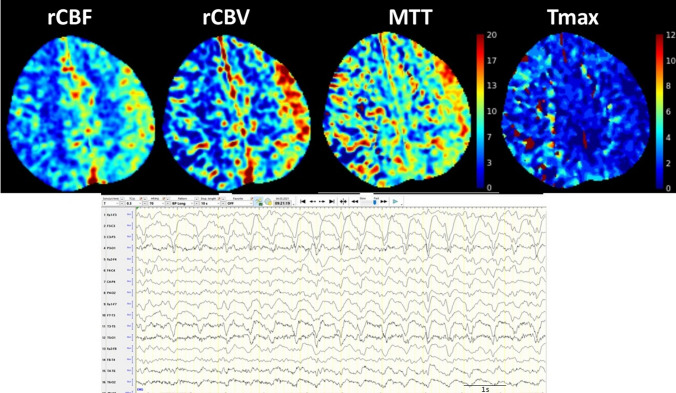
A 78-year-old patient with a history of structural epilepsy following a left middle cerebral artery (MCA) ischemic stroke presented with tonic–clonic status epilepticus, followed by aphasia and right-sided hemiparesis. Computed tomography perfusion (CTP) imaging demonstrated hyperperfusion localized to the anterior, lateral, and posterior MCA territories on the left, with increased cerebral blood volume (CBV) and cerebral blood flow (CBF). EEG performed the following day revealed recurrent seizures lateralized to the left hemisphere, consistent with nonconvulsive status epilepticus (NCSE)
